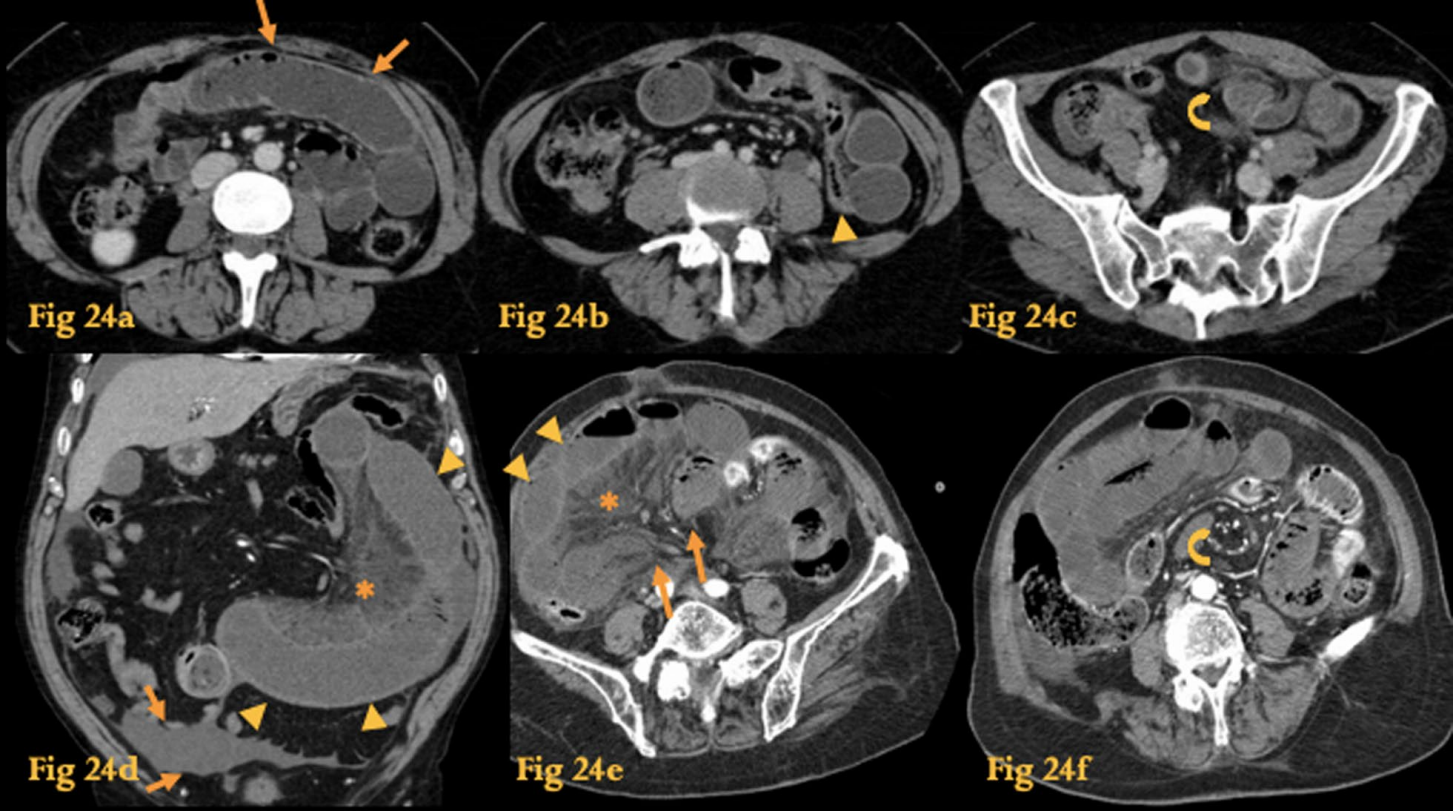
Internal hernia. Abdominal CT of a patient with a left paraduodenal internal hernia there is no fat between the fluid-filled dilated small bowel loop and the anterior abdominal wall (arrows on a). The small bowel loops are seen lateral to the descending colon with medial deviation of the colon (arrowhead on b). There is twisting of the bowel loops and mesentery—whirl sign (curved arrow on c). Another patient with CT findings of a right paraduodenal internal hernia fluid-filled dilated loop of small bowel with a U/C-shape configuration which represents a closed loop obstruction (d). Both ends of a fluid-filled distended closed-loop taper fusiformly, in a similar way to a beak—the beak sign (arrows on e). There is also twisting of the mesenteric vessels at the mesenteric root—whirl sign (curved arrow on f). The fact that there is diminished enhancement of the bowel wall (arrowheads), densification of the mesentery (asterisk) and free fluid (arrows) suggests strangulation (d)
